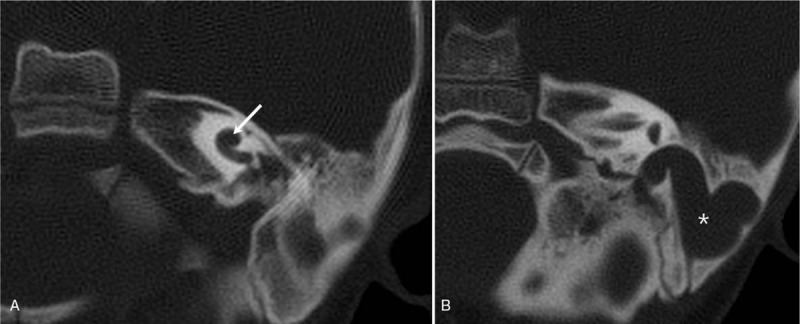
Radiographic finding (axial computed tomography) from the left ear of the patient demonstrating characteristics of CHARGE syndrome. A. the cochlear is dysplastic (arrow). B. the sigmoid sinus shows engorgement (asterisk).
